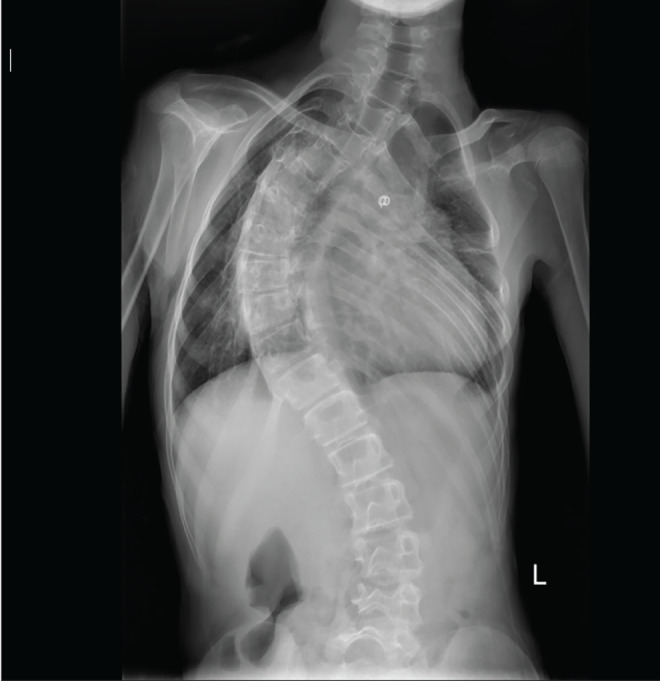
Chest X-ray revealing advanced scoliosis of the patient.
